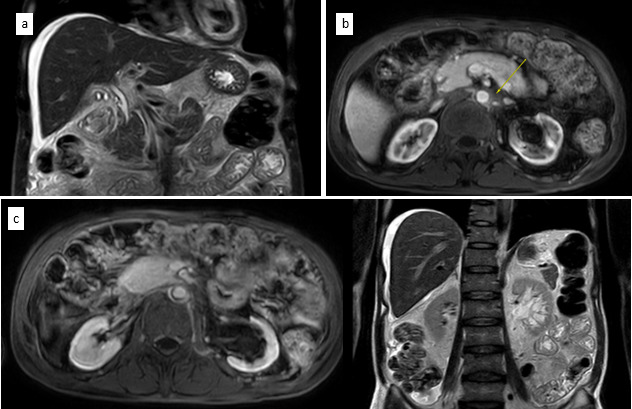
MRI abdomen images. (a) Mild ascites. Biliary tree not dilated. (b) Soft tissue thickening around left post-aortic region. (c) Left renal lymphangiectasia.
